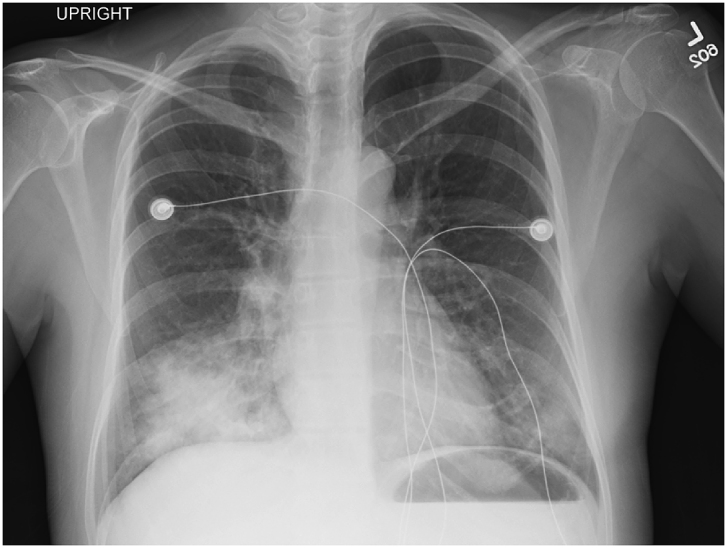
Chest x-ray on hospital day 1 showing right middle and left lower lobe consolidation.
